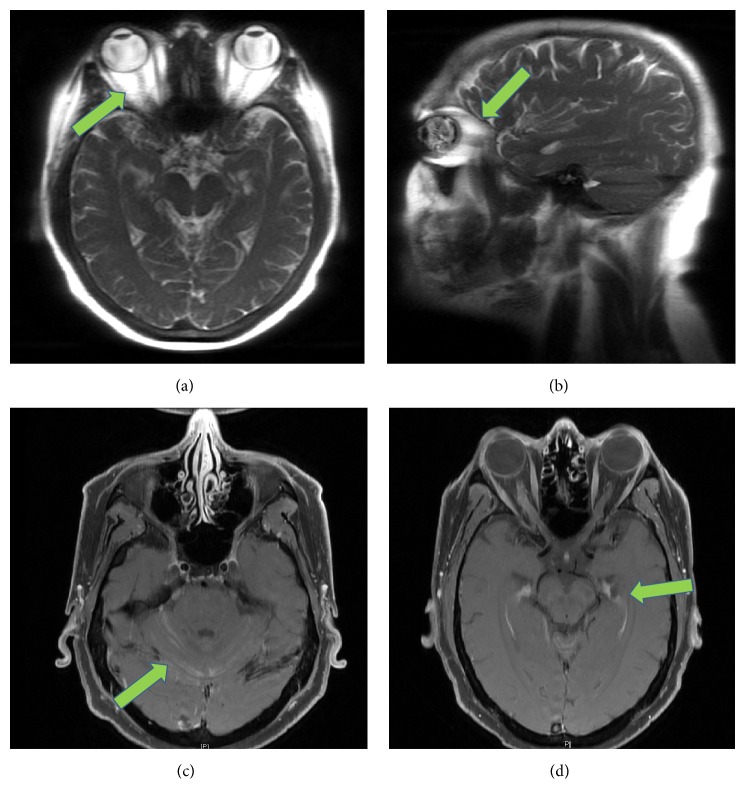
MRI of the brain and orbits. Images show asymmetric enhancement of the right optic nerve sheath (panels (a) and (b)). Arrow denotes greater enhancement in the right optic nerve sheath compared to the left optic nerve sheath, consistent with his clinical presentation. Patient also had bilateral cerebellar surface enhancement (panel (c)) and bilateral trigeminal nerve enhancement (panel (d)).
